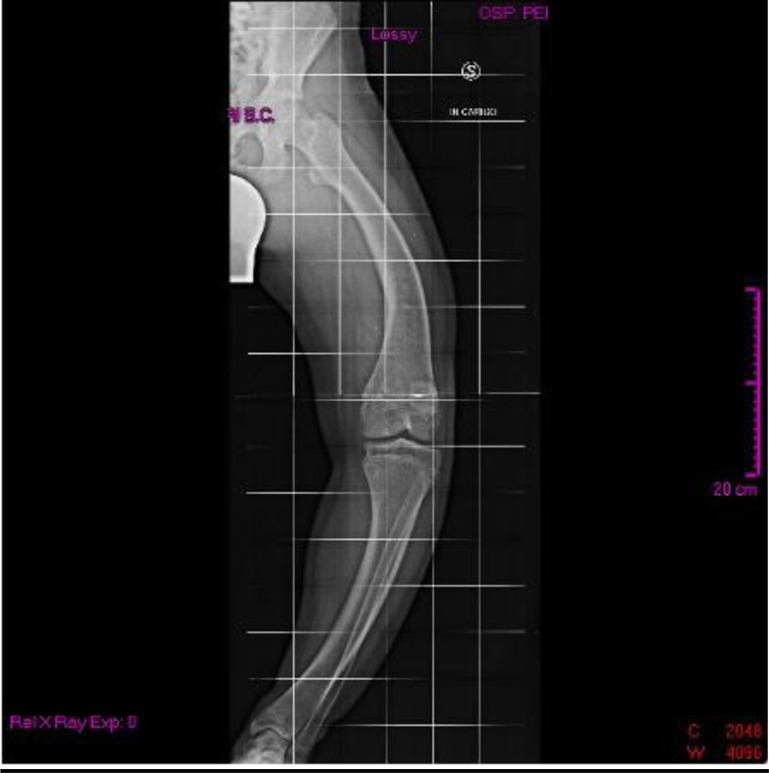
X-ray evaluation of the left leg.
